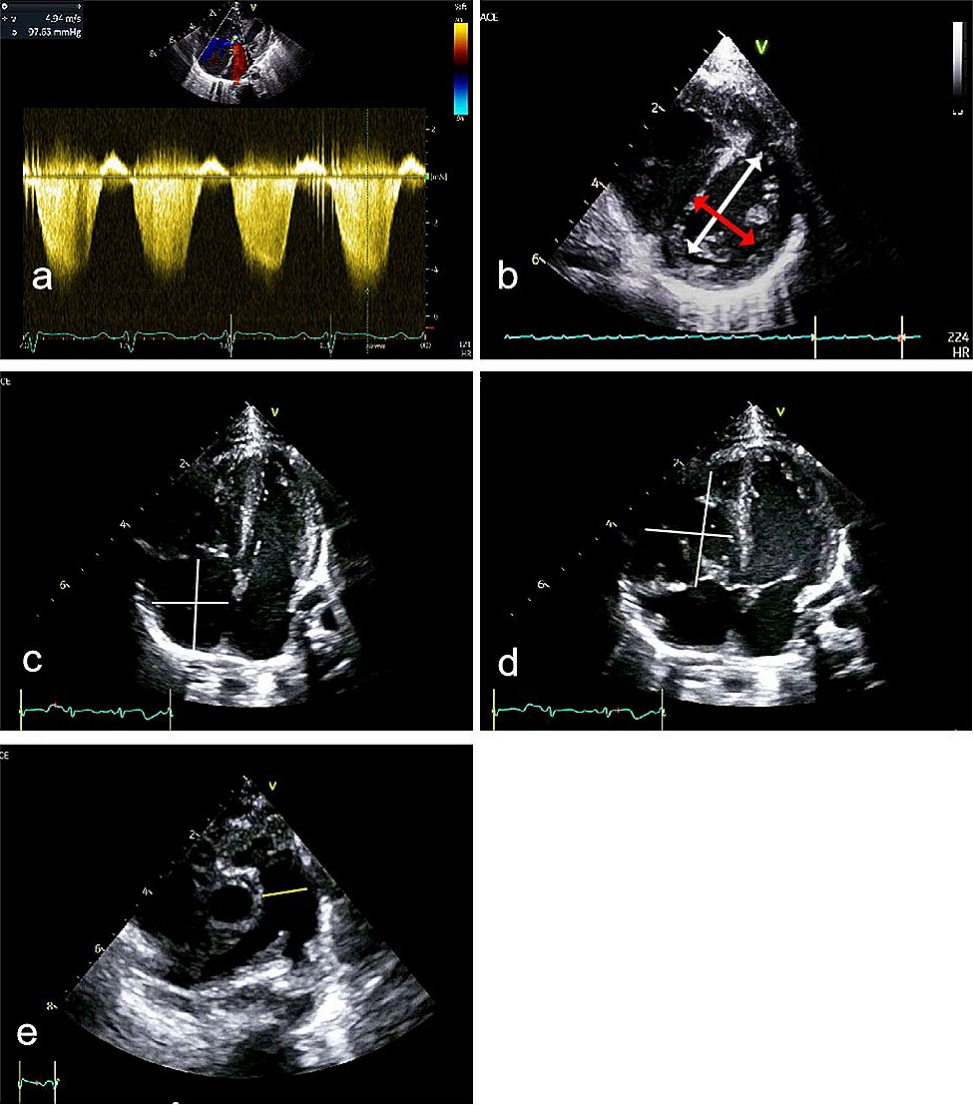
Measurement of the traditional ultrasonic indices. (a) tricuspid regurgitation jet velocity was measured using continuous doppler; (b) The white line represents “D1” and the red line represents “D2” ,left ventricular eccentricity index(LVEI) was obtained through D1 / D2 calculations; (c-d) The inner diameter of right atrium and right ventricle were measured in the four-chamber section of the apex; (e) The inner diameter of pulmonary arteries were measured in the short axis of the aorta
